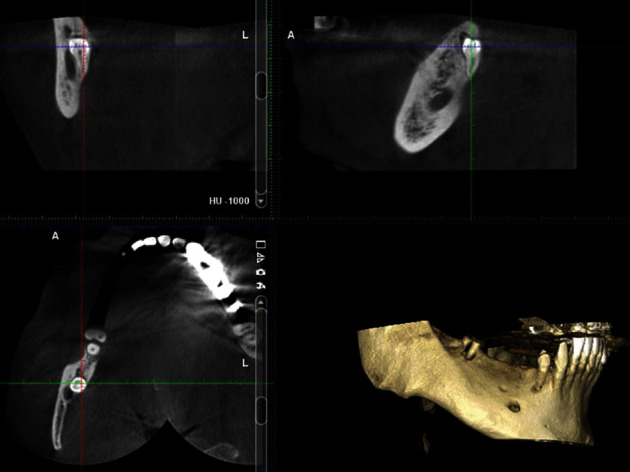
Preoperative CBCT view (coronal, sagittal, axial, and 3D reconstructed section).
